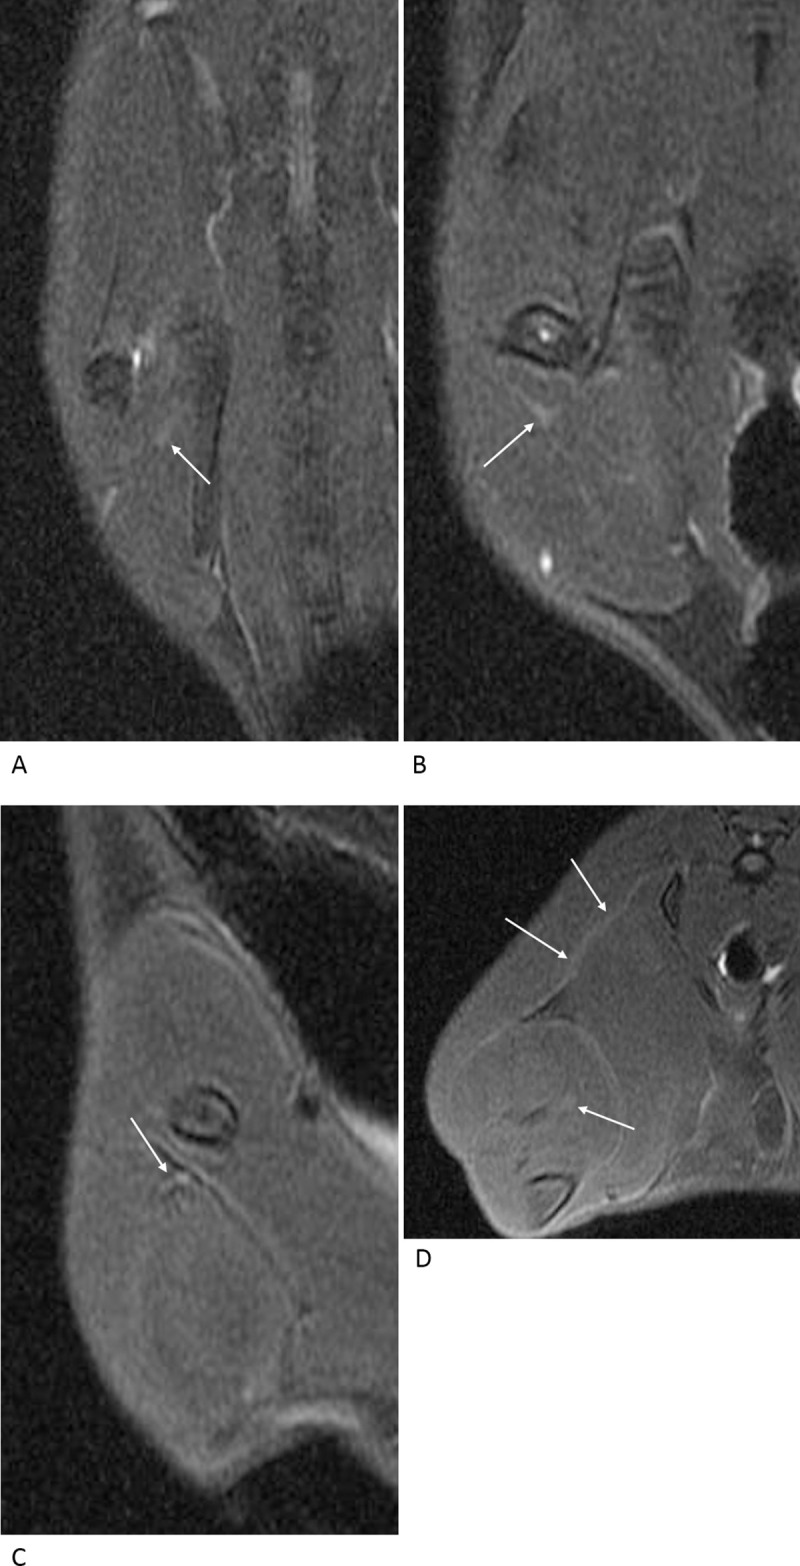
Normal nerve and muscle before surgical intervention. On the oblique coronal (A-C) and axial (D) fat-suppressed T2-weighted images, the expected locations of the left sciatic nerve are indicated by the arrows. However, the structures are not clearly distinguished from the intermuscular fascia. The calf muscles are isointense to the adjacent muscles.
